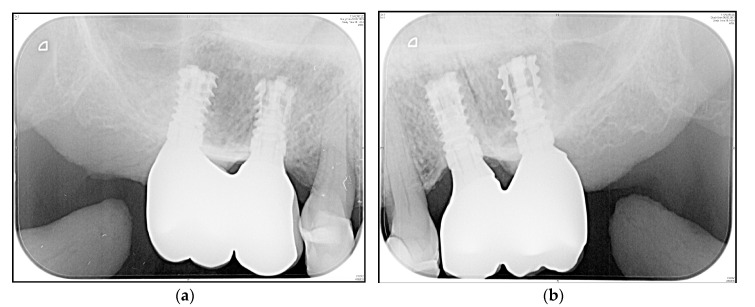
Posterior maxilla of a rehabilitated 62-year old woman at 3.5-year follow-up (Figure 6). (a) Standard periapical radiogram implants i16 and i15; (b) Standard periapical radiogram implants i25 and i26.
